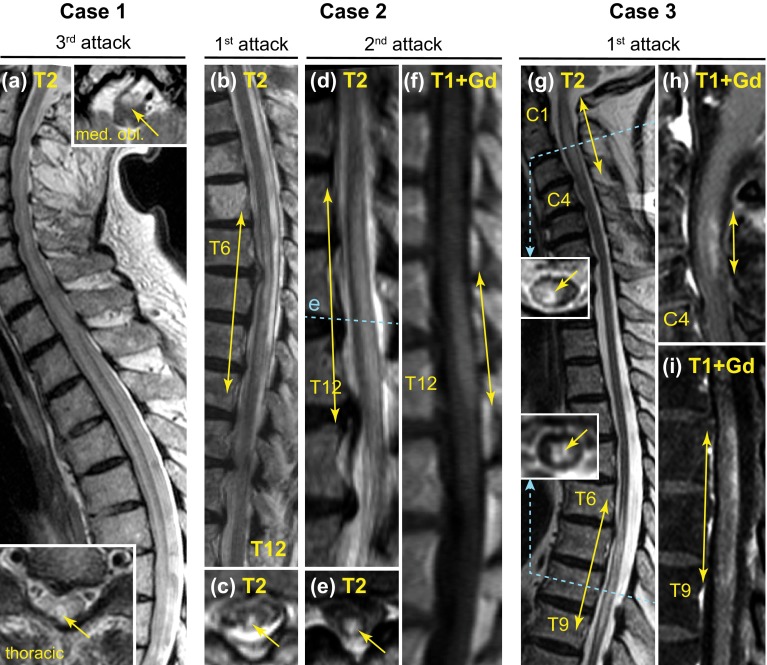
MRI scans of case 1–3 showing longitudinally extensive spinal cord lesions.* Yellow solid arrows* indicate extensions of lesions. The lesion extended throughout the entire myelon and there were also lesions in the brainstem in case 1 (a) (*upper inset* in a). *Blue dashed lines* in the sagittal images indicate the levels of related axial scans. *med. obl.* axial scan at level of medulla oblongata, *C* and *T* indicate the respective cervical and thoracic vertebrae; *T2* T2 weighed MRI sequence, *T1* T1 weighed MRI sequence, +*Gd* gadolinium-enhanced sequence
